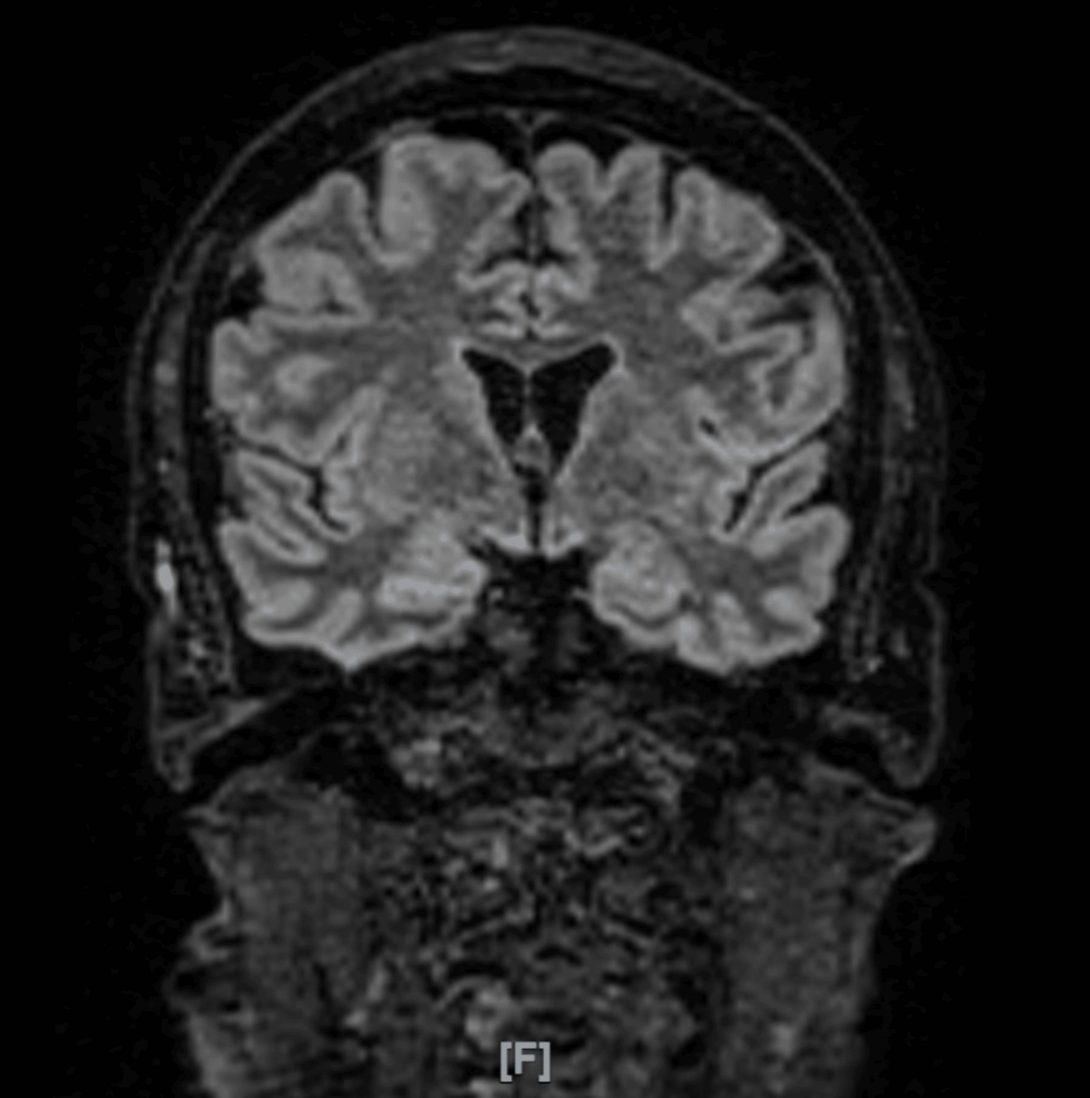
Coronal T2 FLAIR MRI of the brain showing no acute intracranial abnormalities FLAIR: Fluid-attenuated inversion recovery
